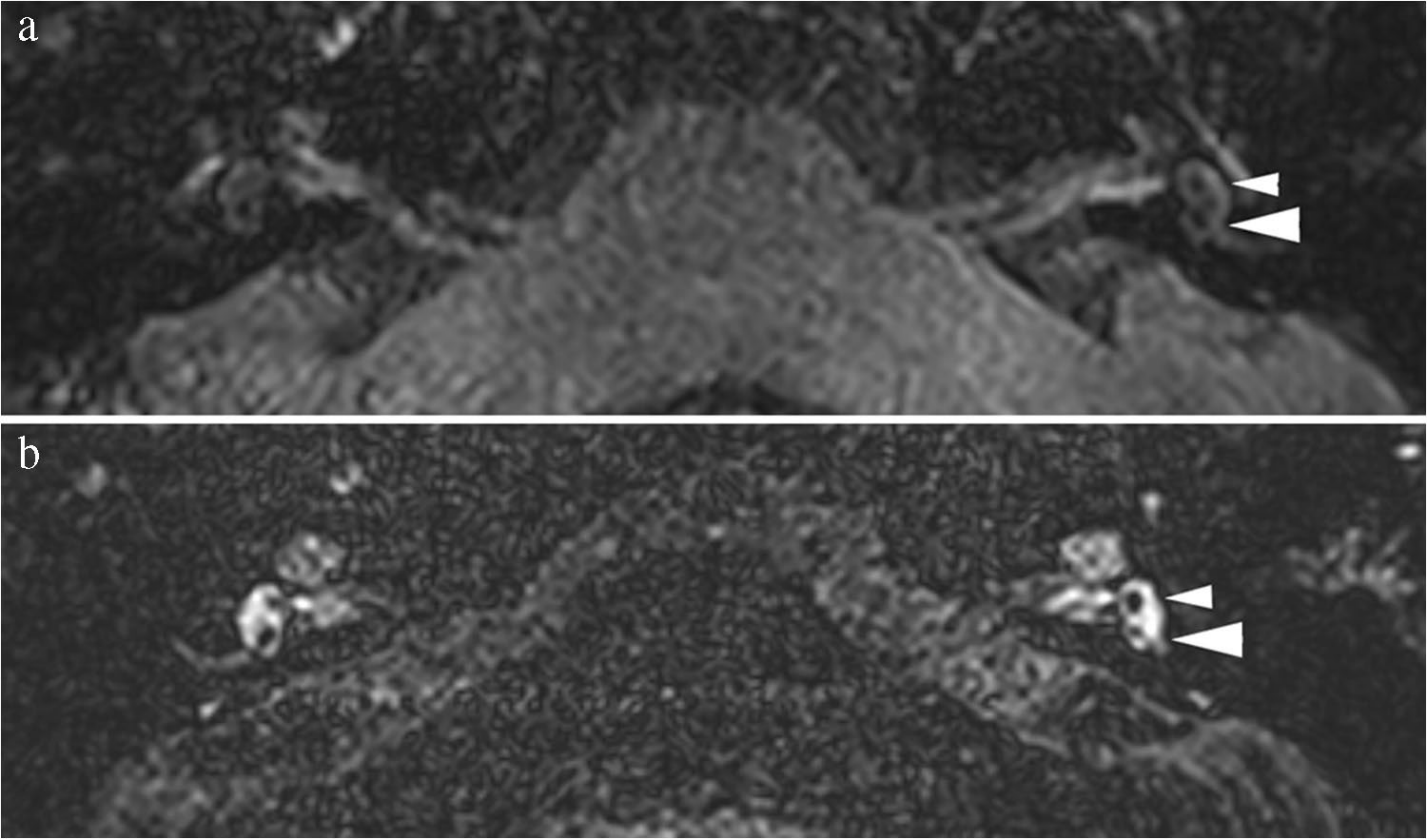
Sixty-one-year-old male with attacks of vertigo, left-sided hearing loss associated with tinnitus and pressure sensation, clinically categorized as definite MD. Imaging performed 4 h after IV administration of a single dose of Gd. a Axial 3D TSE FLAIR image at the level of the vestibule. Normal aspect of the saccule (small arrowhead) and utricle (large arrowhead) on the left side. Difficult visualization of the saccule on the right side. No signs of vestibular hydrops. b Axial 3D SPACE FLAIR at the level of the vestibule. No abnormalities on the right side, with a saccule appearing smaller than the utricle. On the left side, size relation between saccule and utricle is inverted with a saccule (small arrowhead) being larger that the utricle (large arrowhead) without being confluent: vestibular EH grade I on the left side. Note the much higher signal intensity and sharper delineation of the perilymphatic and enlarged endolymphatic spaces on the 3D SPACE FLAIR compared to the 3D TSE FLAIR, both at the level of the cochlea and at the level of the vestibule. No cochlear hydrops was seen in this patient (not shown)
